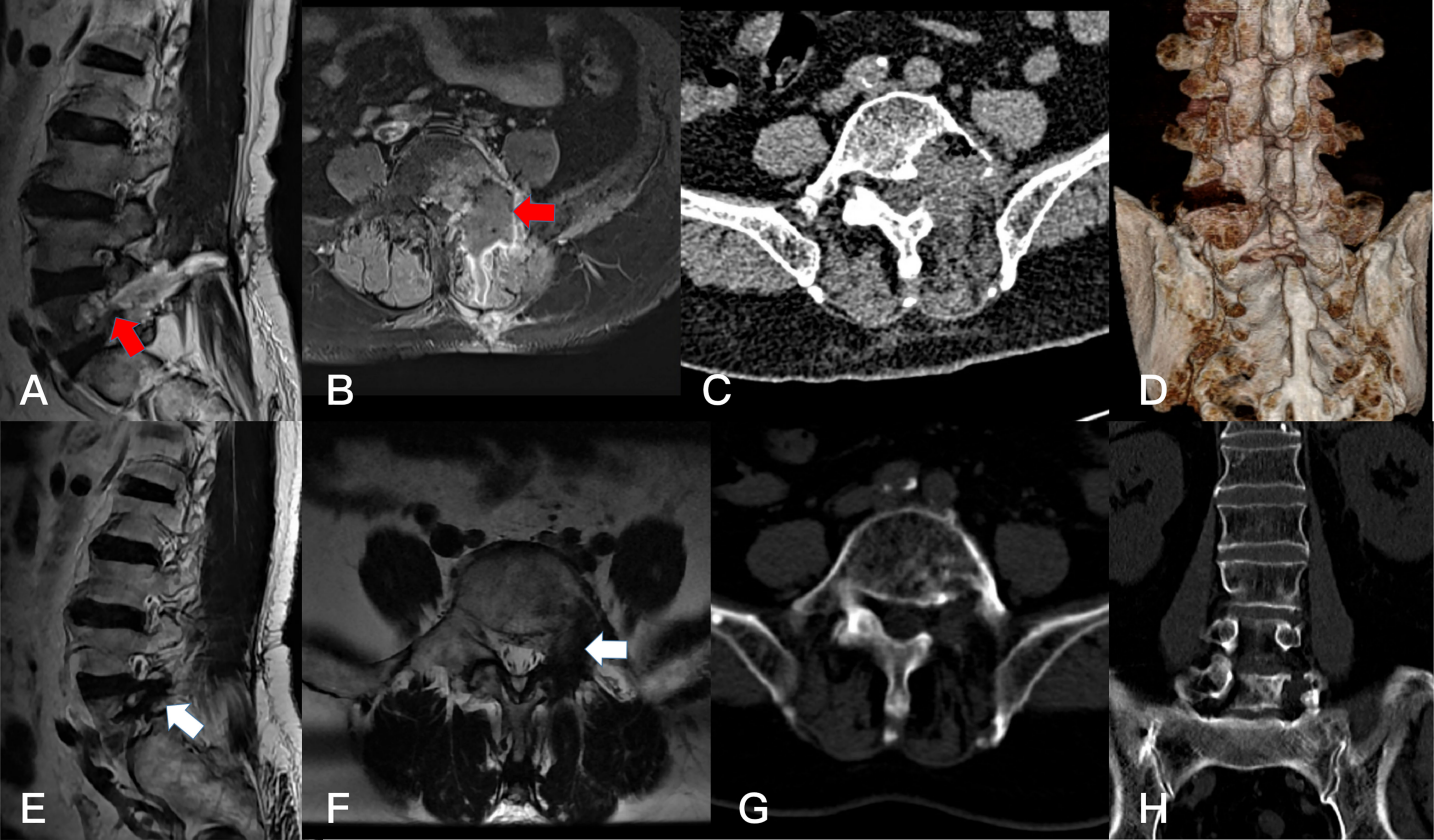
Postoperative T1-weighted enhanced MRI on sagittal (A) plane, and axial (B) palne, and the axial (C) and 3D-CT (D) scan. Resection of the dumbbell acute lymphocytic leukoma (B cell) could not be identified after 1 months (arrows). Postoperative T1-weighted enhanced MRI on sagittal (E) plane, and axial (F) palne, the CT scan of the axial (G) plane and coronal (H) plane after 2 years, showing the resection of the dumbbell tumor could not be identified (arrows).
